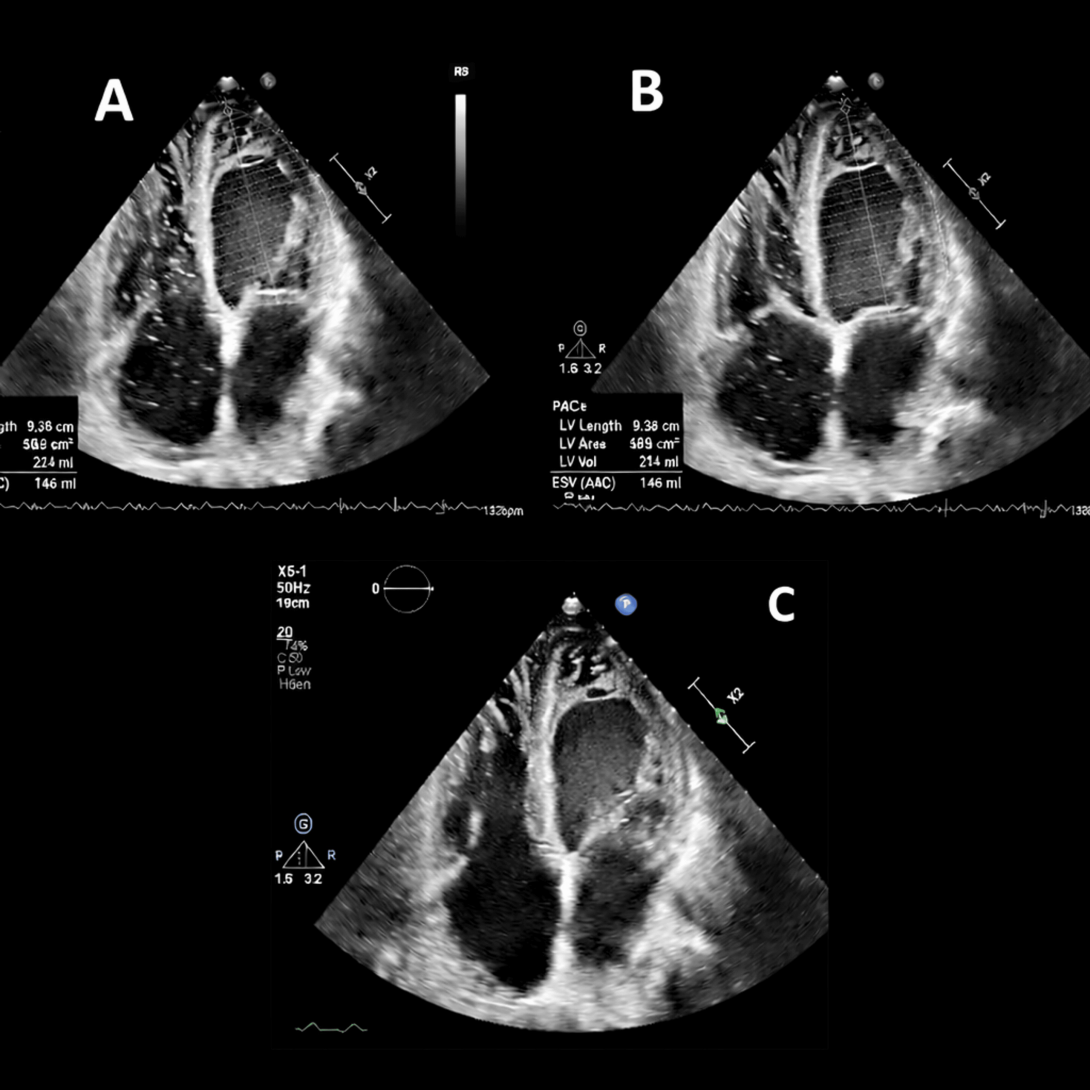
Transthoracic echocardiography (TTE) images from Case 3. (A-B) Apical four-chamber views demonstrating a severely reduced left ventricular ejection fraction (LVEF) of 4.46%. (C) Apical view showing prominent left ventricular (LV) trabeculations consistent with noncompaction cardiomyopathy.
